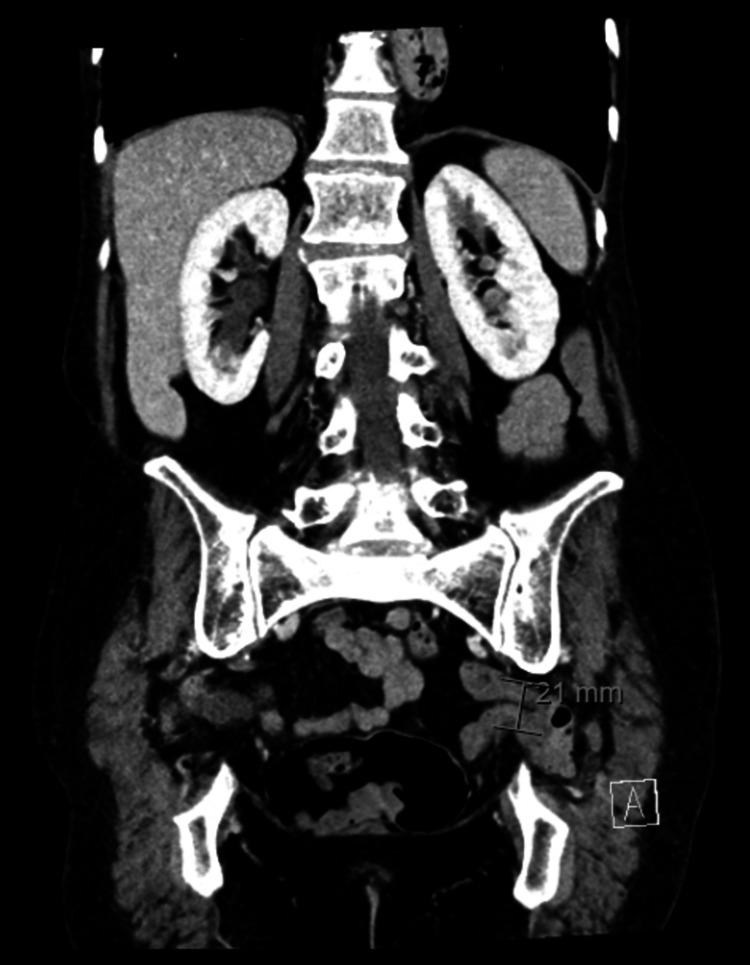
Coronal CT image demonstrating the same left-sided sciatic hernia with a maximum dimension of 21 mm.
